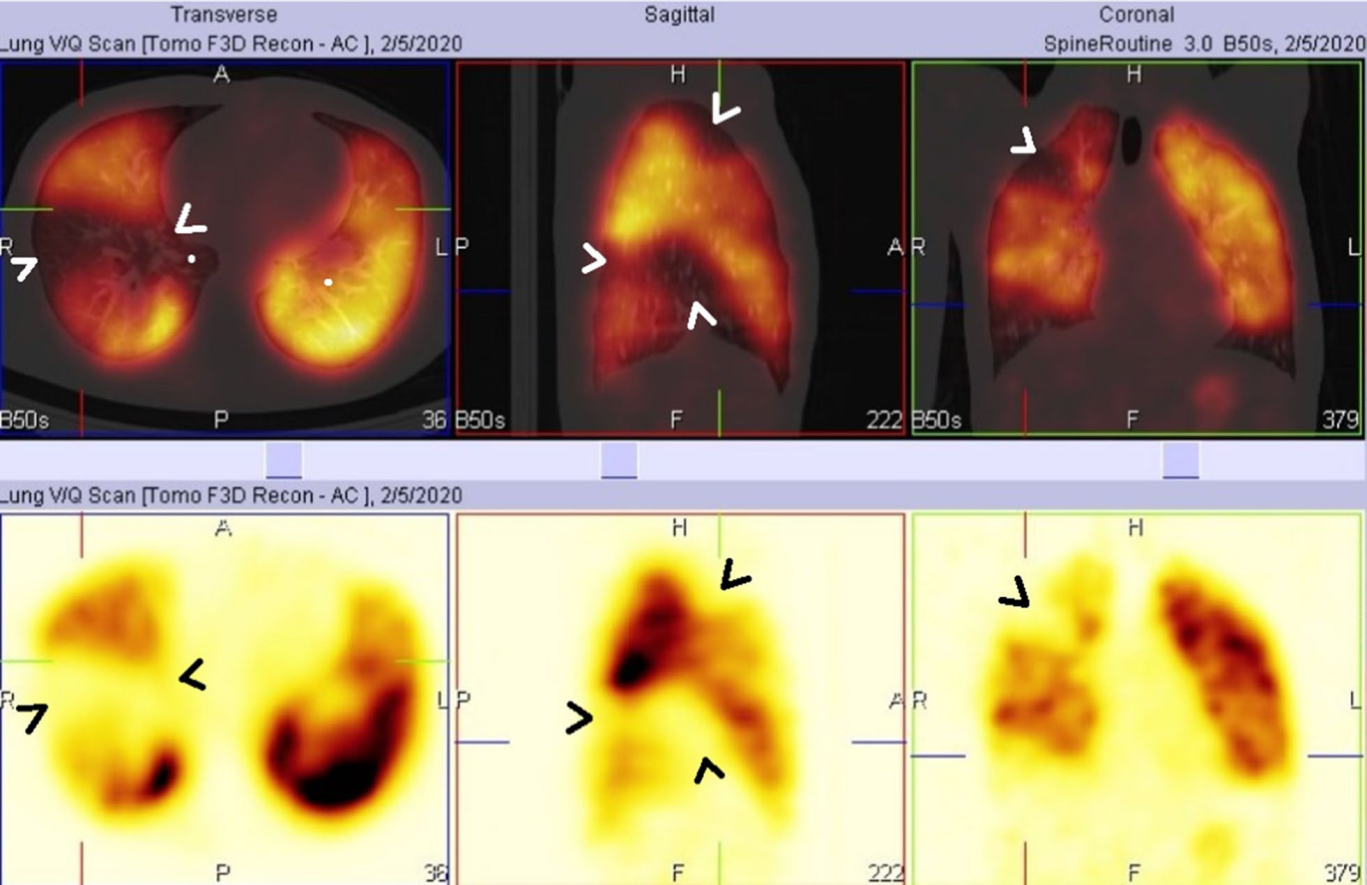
Axial and reconstructed views of SPECT-CT slices show multiple right lung middle lobe (medial and lateral segmental), upper lobe anterior segment, lower lobe anterobasal, laterobasal, and posterobasal segment perfusion defects (arrow heads)
